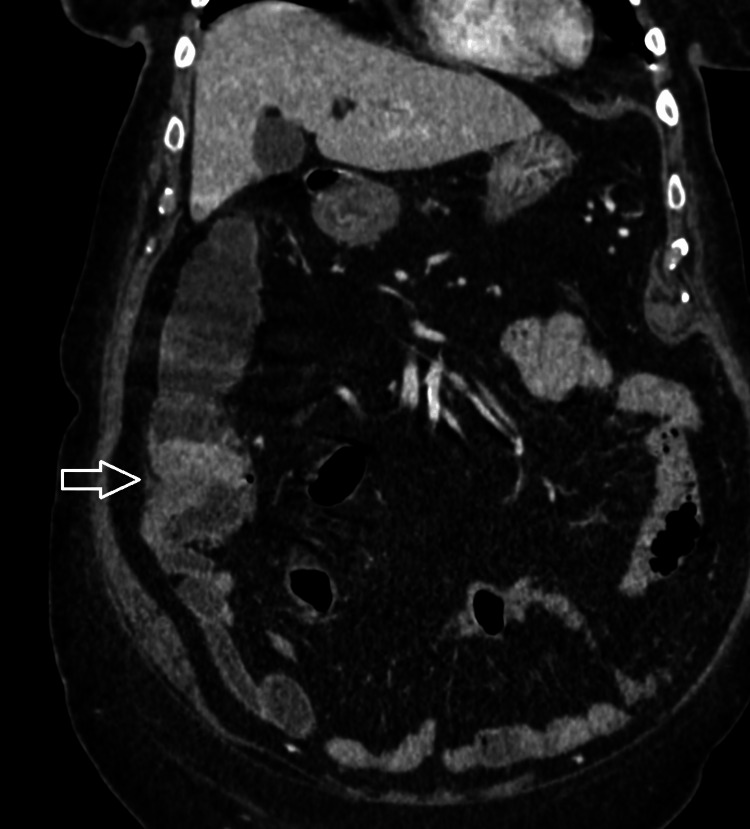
Sagittal view of caecal mass on computed tomography
